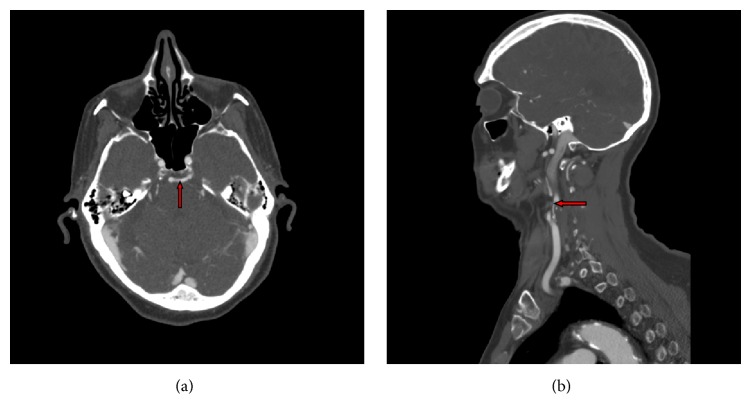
(a) A CT angiogram showing a persistent trigeminal artery arising from the left cavernous internal carotid artery and supplying the mid-distal basilar artery (red arrow). (b) A CT angiogram of the neck revealing the severely stenosed left internal carotid artery (red arrow).
